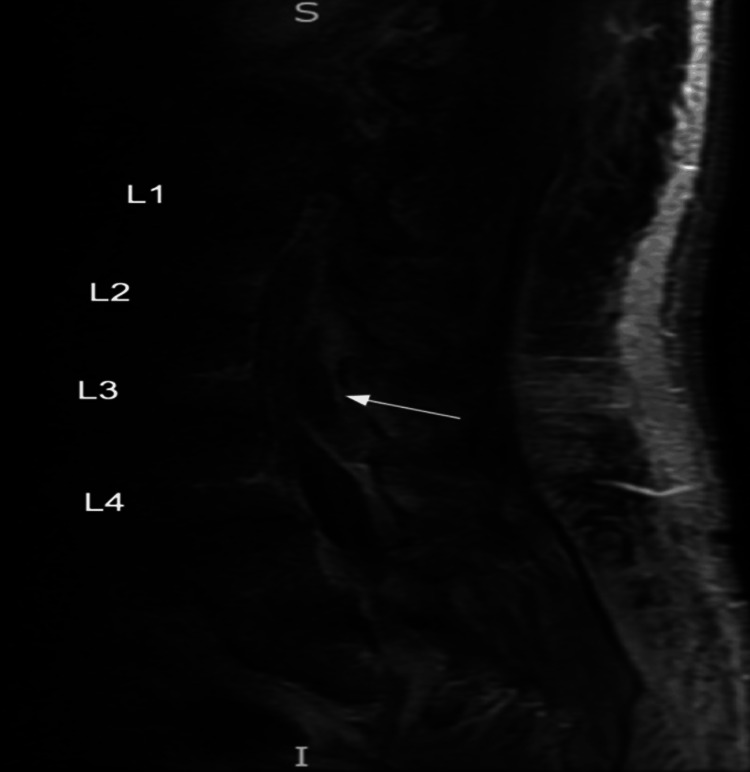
MRI imaging of an epidural abscess (white arrow) Magnetic resonance imaging (MRI) sequence T2 with gadolinium contrast of the lumbar spine exhibits a T2 hyperintense rim-enhancing collection in the dorsal epidural space at the L2-L4 level, with a thickness of 8mm. This results in the anterior displacement of the cauda equina nerve roots, accompanied by a partial collapse of the thecal sac.
